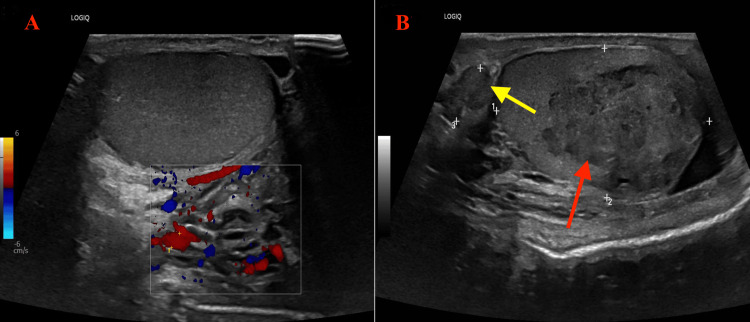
Scrotal ultrasound with color Doppler A: Normal right testis with normal vascularity. B: Heterogeneous and ill-defined mass lesion in the left testis, occupying the mid and lower poles (red arrow).
